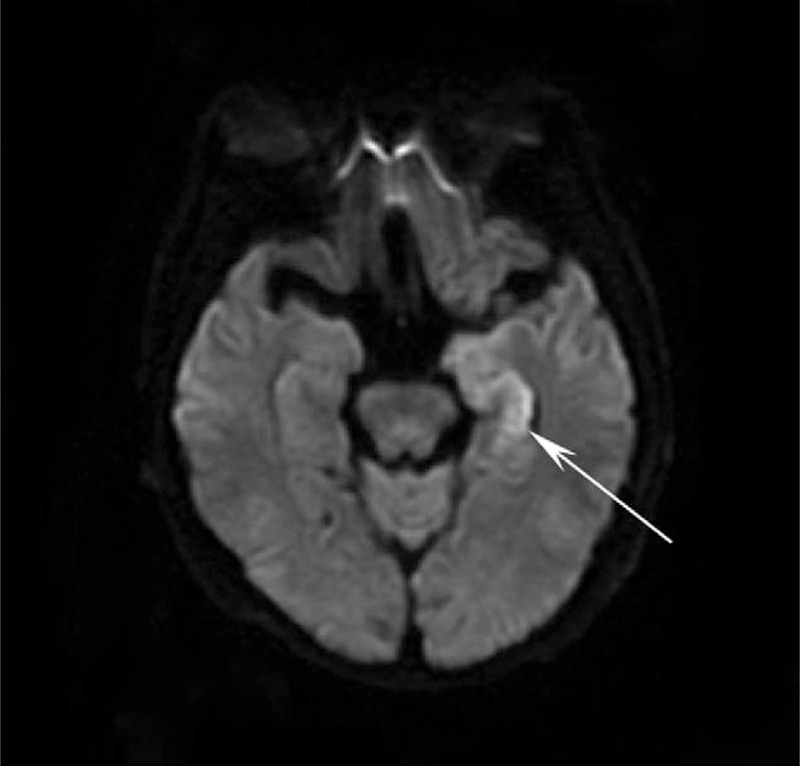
DWI. Cranial MRI images revealed left hyperintensity of the hippocampus on the DWI sequence. DWI = diffusion-weighted imaging, MRI = magnetic resonance imaging.
